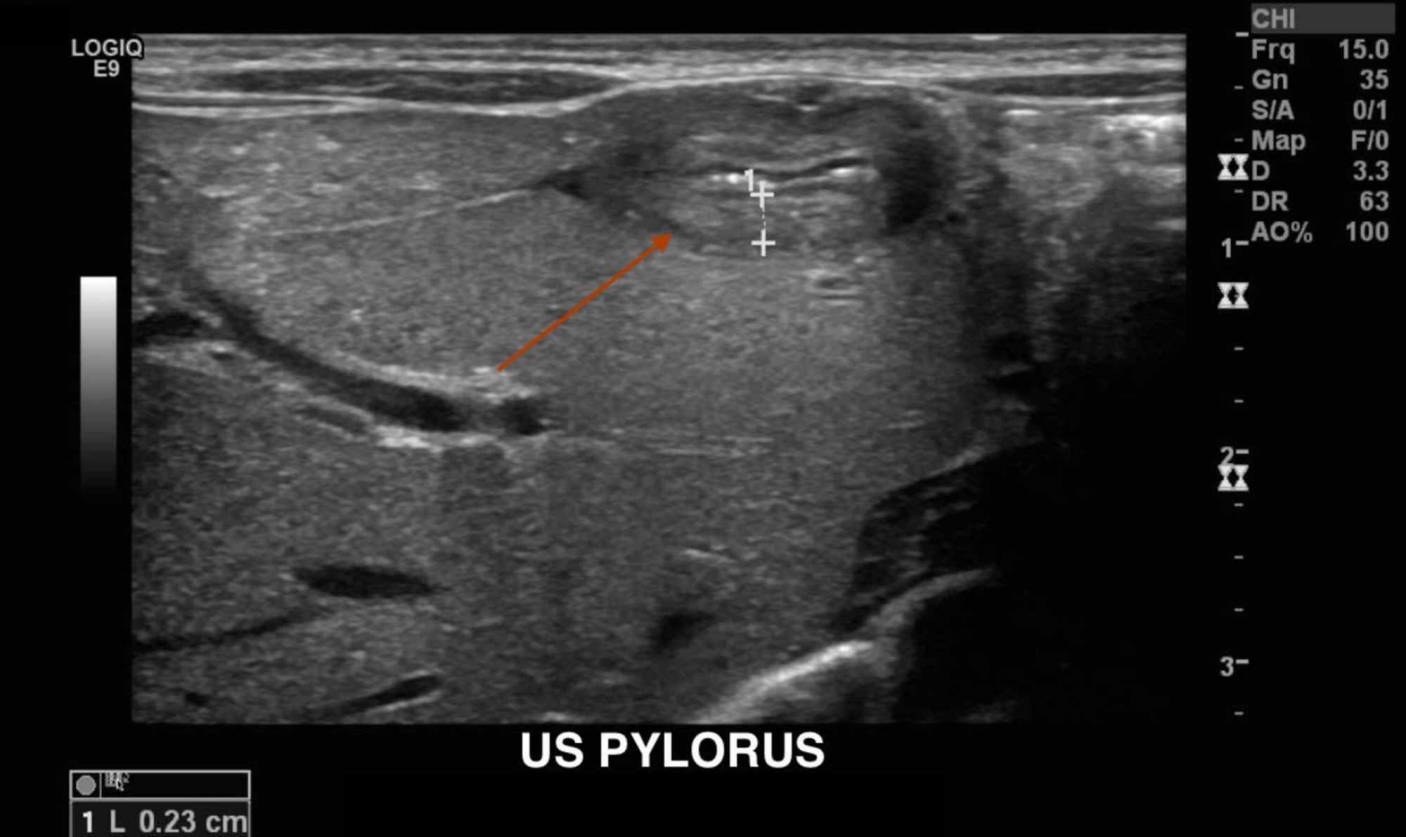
Ultrasound of the pylorus
